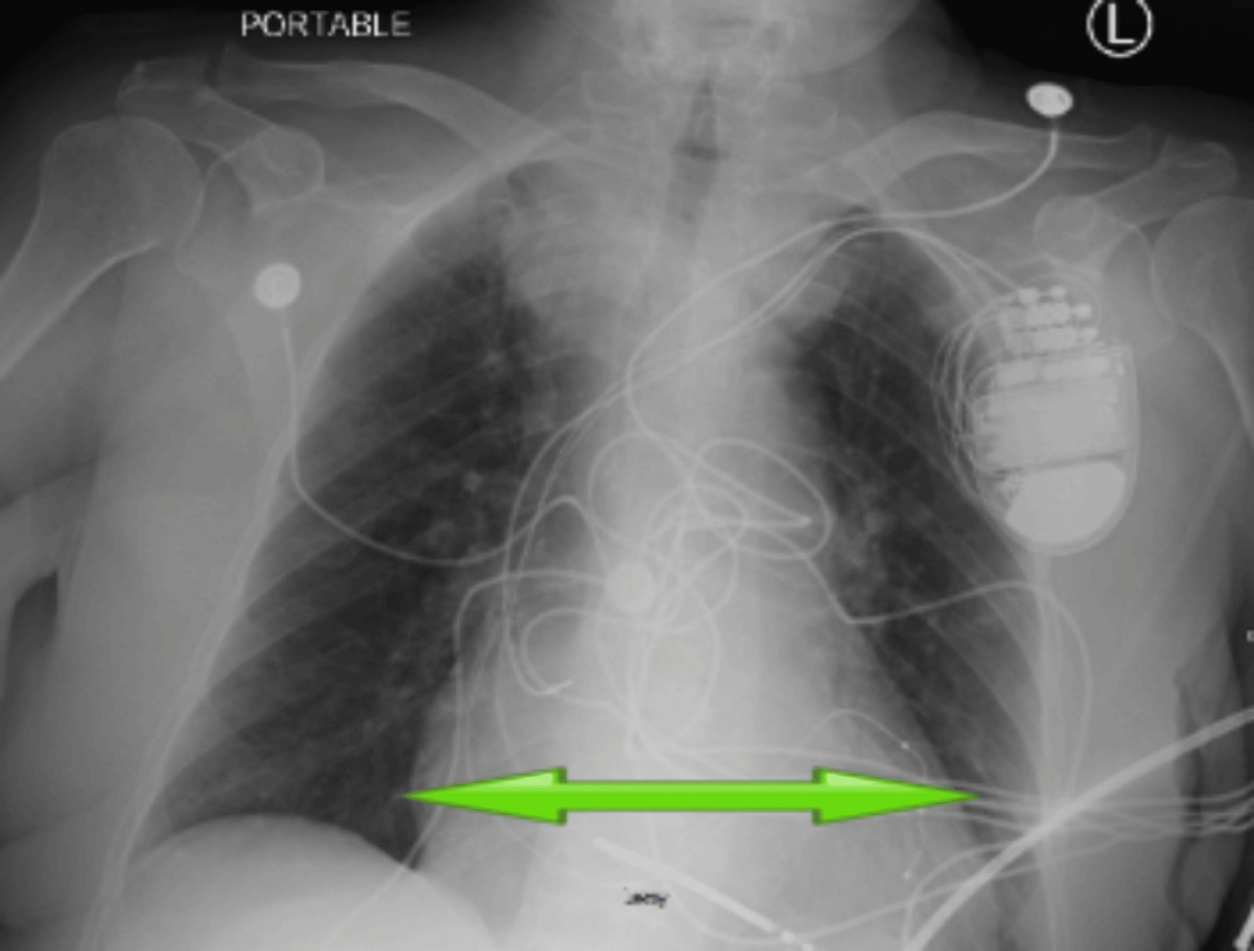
Chest X-ray on admission showing cardiomegaly
